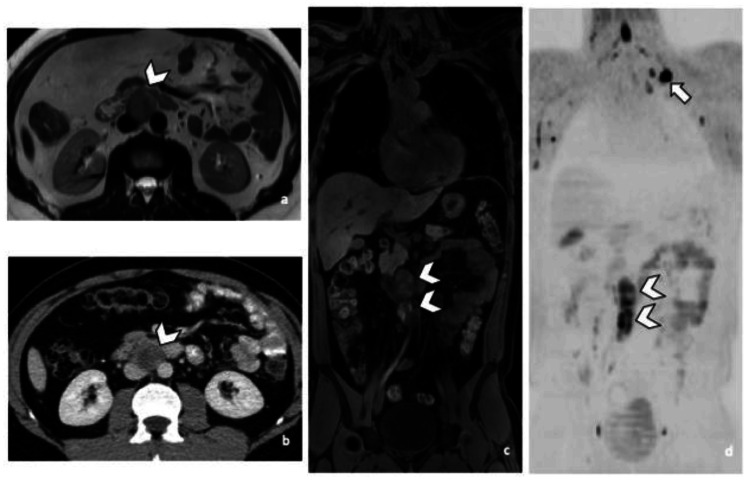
MRI and CT imaging of retroperitoneal lymph node metastases in a patient with non-seminomatous germ cell cancer. Axial T2-weighted MRI (a), axial CT with contrast (b), coronal 3D T1-weighted MRI with fat suppression (c), and diffusion-weighted MRI (d) demonstrating interaorticocaval enlarged lymph nodes (arrowheads) in a 29-year-old man. A left supraclavicular lymphadenopathy is also visible on diffusion-weighted imaging (d, arrow). Image reused from Pasoglou et al. [26] under the terms of the Creative Commons Attribution (CC BY) license.
